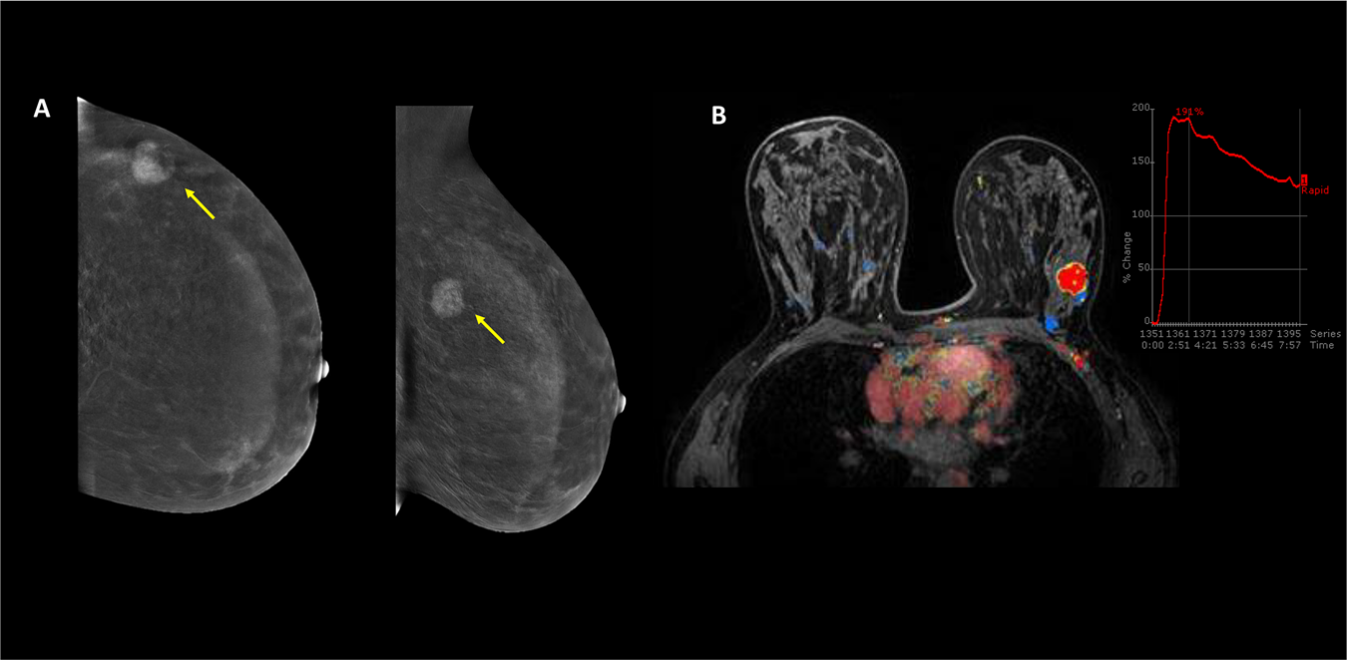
Representative CEM recombined and MR images for a 38-year-old woman with invasive NST carcinoma, grade 3, HR+/HER2− and high-grade DCIS. A Enhancing mass lesion in the left upper outer quadrant (yellow arrows) demonstrating high conspicuity on CC and MLO views and wash-out enhancement pattern (%RSD: −22.5). B Corresponding post-contrast MRI with type III enhancement. CEM, contrast-enhanced mammography; NST, no special type; HR, hormone receptor; HER2, human epidermal growth factor 2; DCIS, ductal carcinoma in situ; CC, craniocaudal; MLO, mediolateral oblique; %RSD, percent relative signal difference
